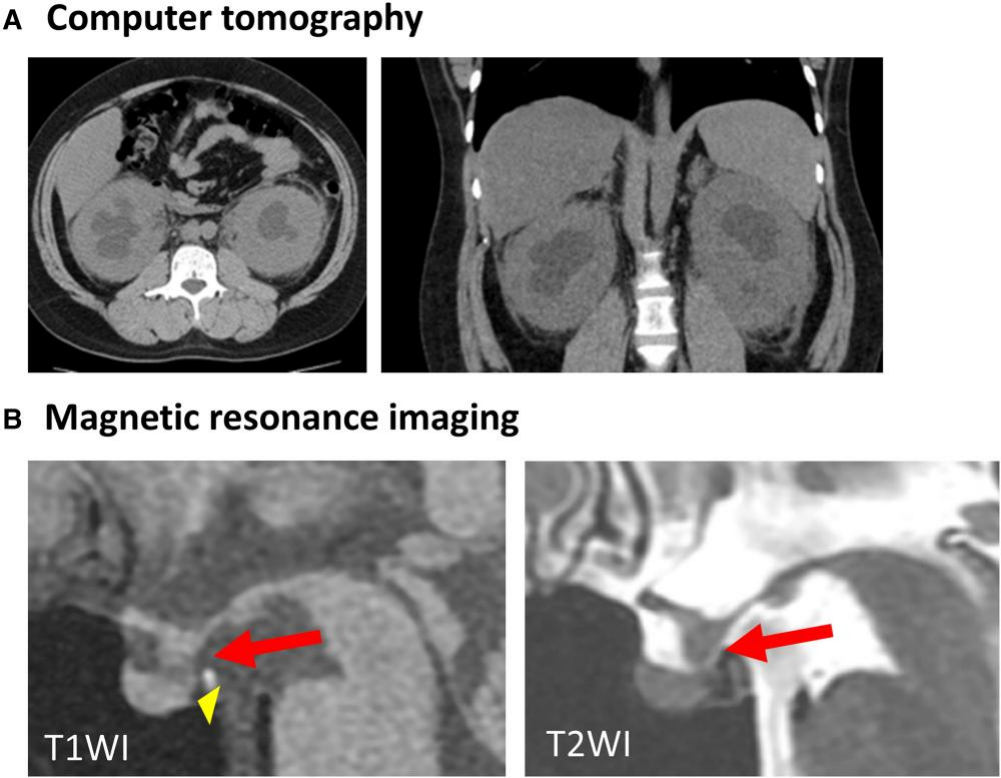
A, Abdominal plain computed tomography before treatment. “Hairy kidney” can be seen in bilateral kidneys. B, Pituitary magnetic resonance imaging before treatment. Pituitary stalk thickness (red arrow) and loss of high-intensity area on T1WI (yellow arrowhead) are apparent.
